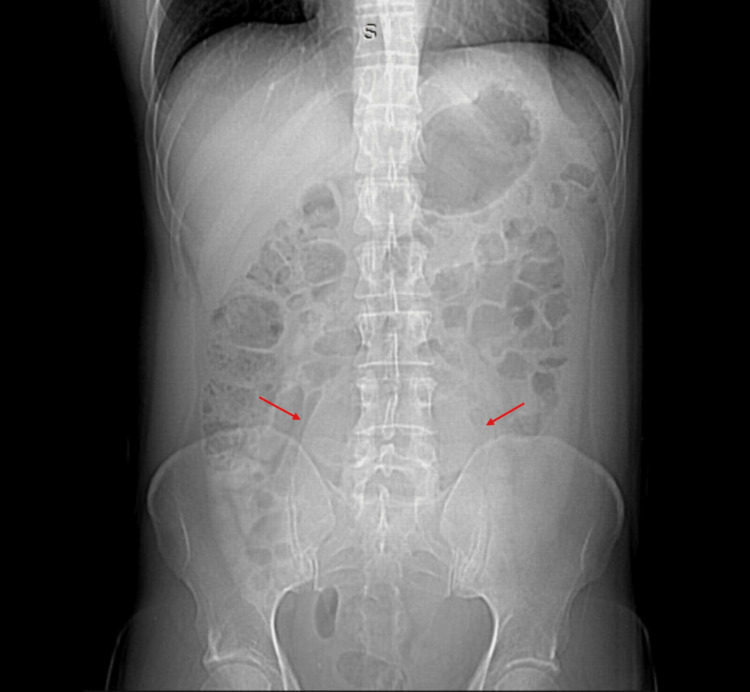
Plain abdominal radiograph showing a pelvic space-occupying lesion displacing the intestinal loops
